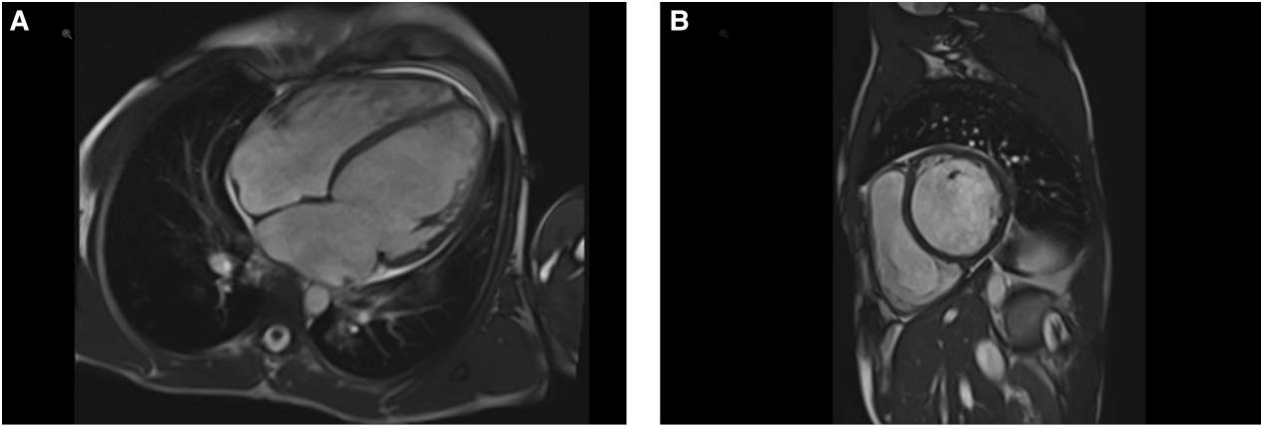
Cardiac magnetic resonance imaging (MRI). (*A*) Four-chamber view. (*B*) Short-axis view.
